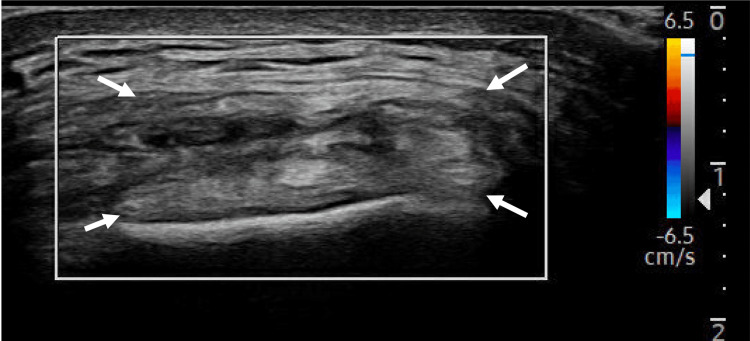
Short-axis view of the distal quadriceps tendon with color Doppler showing no hyperemia within the tendon. Arrows: Quadriceps tendon.
